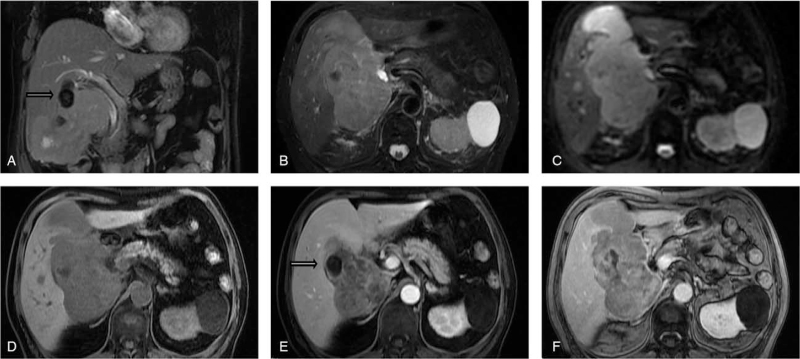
Abdominal magnetic resonance imaging with perfusion-weighted imaging showing a 13.5 × 9.9 cm enlarged gallbladder with gallbladder stones (the largest measured 3.5 × 2.1 cm) and thickened wall, and invasion of surrounding liver. A and B: T2-weighted images, C: DWI image, D: water + c phase, E: water phase, F: out of phase image, and arrow: gallbladder stones. DWI = dippusion-weighted imaging.
